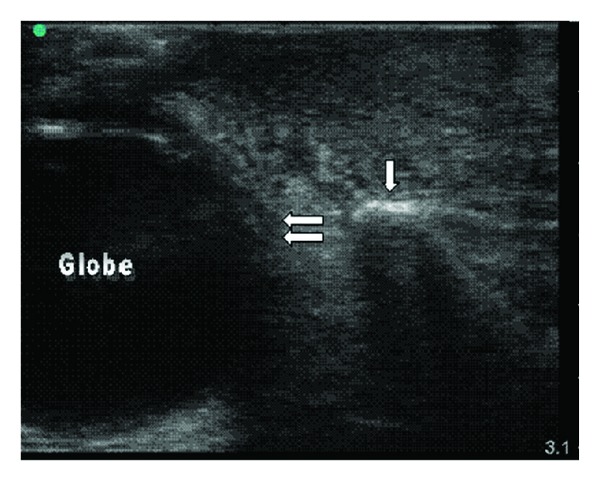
Bedside ultrasound of the case patient using linear probe, transverse view. The hyperechoic area labeled with a single arrow represents the maxilla. There is an area of cellulitis within the subcutaneous tissue above the maxilla which is distinguished by the hypoechoic streaks of fluid interrupting the normal tissue organization. The edge of the globe of the eye is labeled with double arrows.
